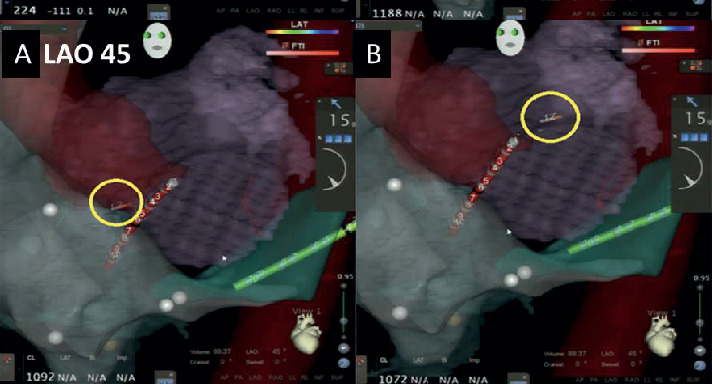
Direct visualization (yellow circle) of the needle tip on the electroanatomical mapping system (Left Anterior Oblique Projection): needle tenting on the fossa ovalis (A) and in the left atrium (B). FAM of right atrium (RA) merged with the 3D reconstruction of a preacquired CT scan and two decapolar catheters in His and coronary sinus (CS) position. Ao: aorta.
